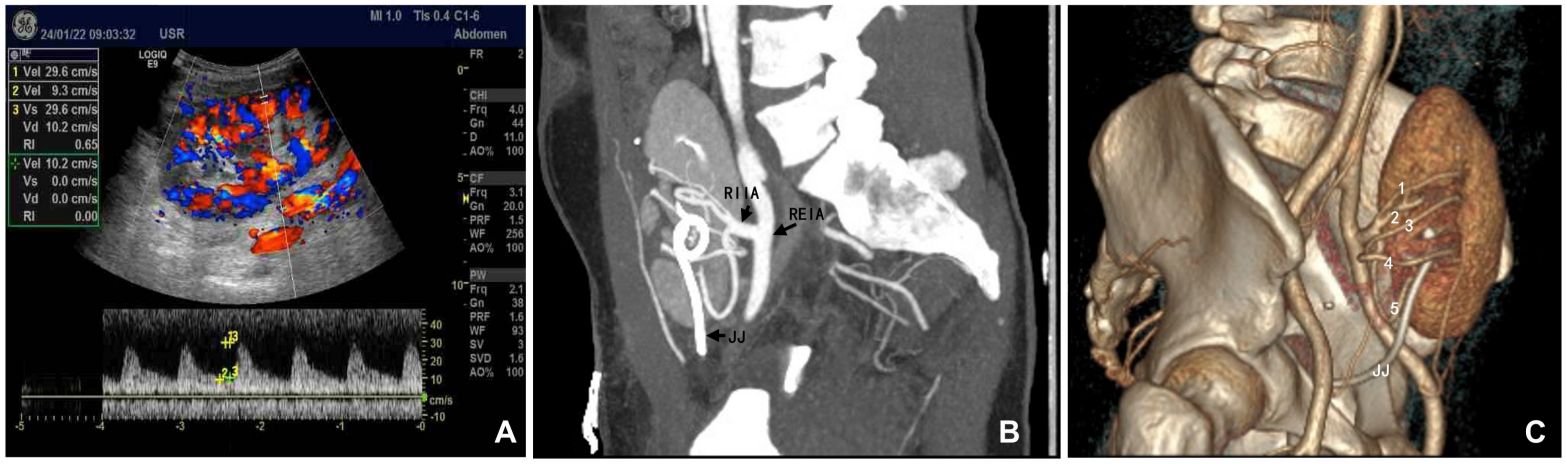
Postoperative evaluation of the transplanted kidney. (A) Color Doppler ultrasound performed on the day of surgery demonstrating robust blood flow within the kidney graft. (B, C) Computed tomography angiography performed 2 weeks post-transplantation, clearly delineating all five renal arteries. RIIA, recipient internal iliac artery trunk; REIA, recipient external iliac artery; JJ, double-J ureteral stent within the transplanted kidney.
